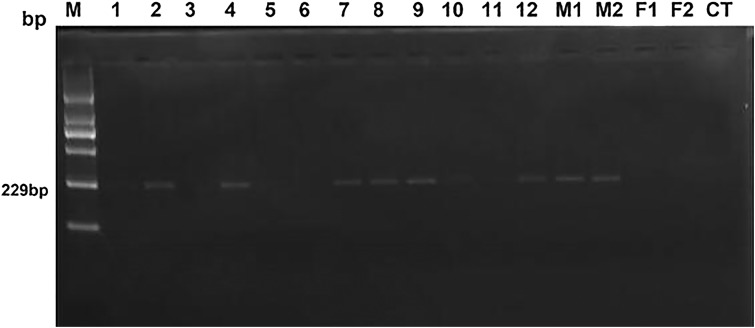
The electrophoresis results of test and control samples by PCR. Lane 1 (M): Molecular weight marker (2,000-bp DNA ladder); bands from bottom to top are listed as 100, 250, 500, 750, 1,000 and 2,000 bp; the size of target bands is 229 bp. Lanes 2 through 13 (No.1–No.12) display samples of 12 female heterosexual twins. Lanes 14 (M1) and 15 (M2) represent positive control. Lanes 16 (F1) and 17 (F2) show negative control. The rightmost lane (CT) is the blank control.
